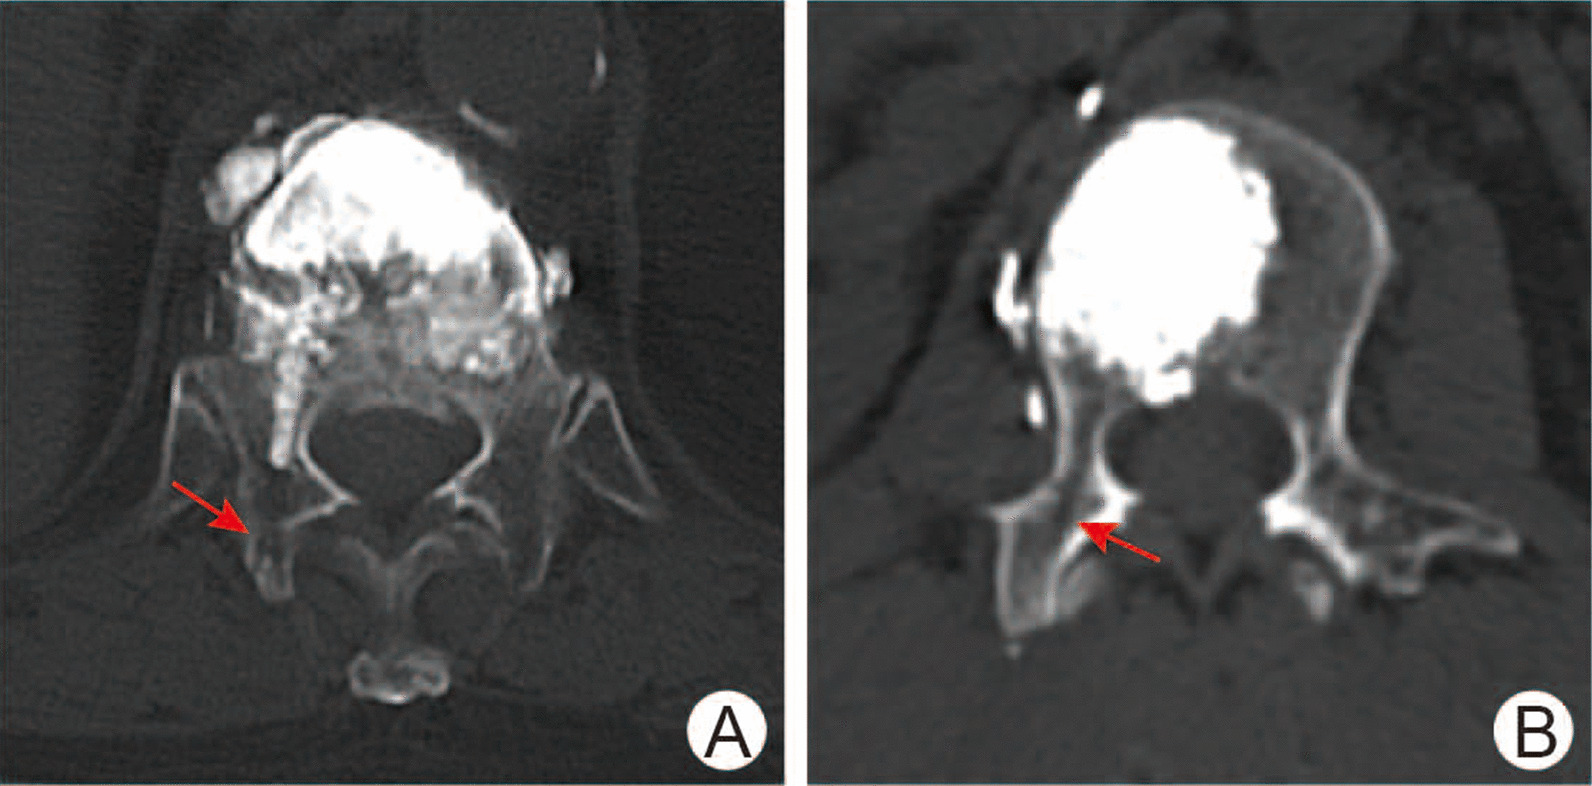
CT transversal view of the trajectory after PVP surgery. A Male, 58 years old, who suffered a T12 vertebral fracture and accepted traditional puncture PVP surgery. B Male, 81 years old, who suffered a L2 vertebral fracture and accepted extra-facet puncture PVP surgery
